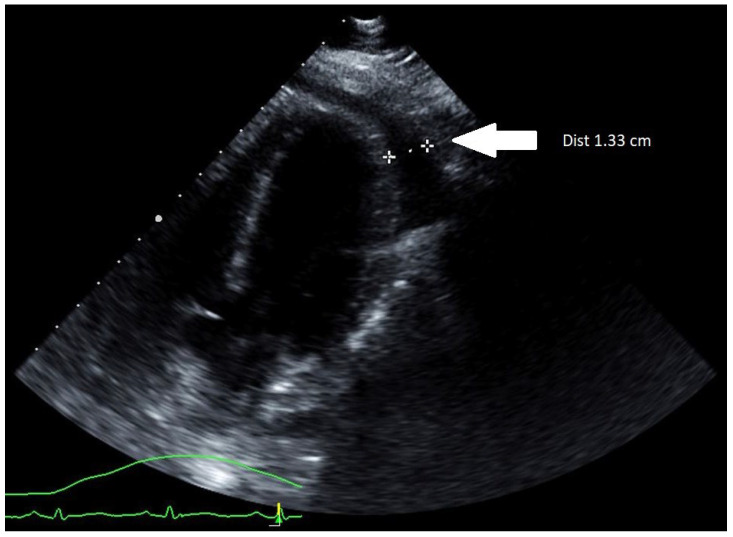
TTE (parasternal long-axis view) showing an increasing pericardial effusion measuring 1.33 cm (marked by two white stars).
